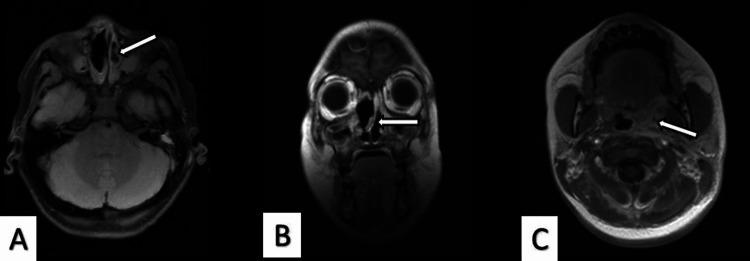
Cranial CT scan findings. (A) Axial image showing ethmoid-maxillary sinusitis in the left nasal cavity (arrow indicating the left maxillary sinus). (B) Coronal image showing mucosal thickening in both maxillary sinuses, bilateral anterior osteomeatal complex obstruction, and septal deviation (arrow indicating the nasal septum). (C) Axial image showing a hypodense collection in the left parapharyngeal region consistent with an abscess (arrow indicating the left parapharyngeal region).
